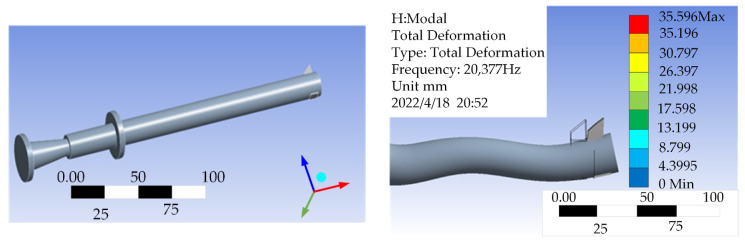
Modal analysis of the two-dimensional ultrasonic amplitude modulation system.
